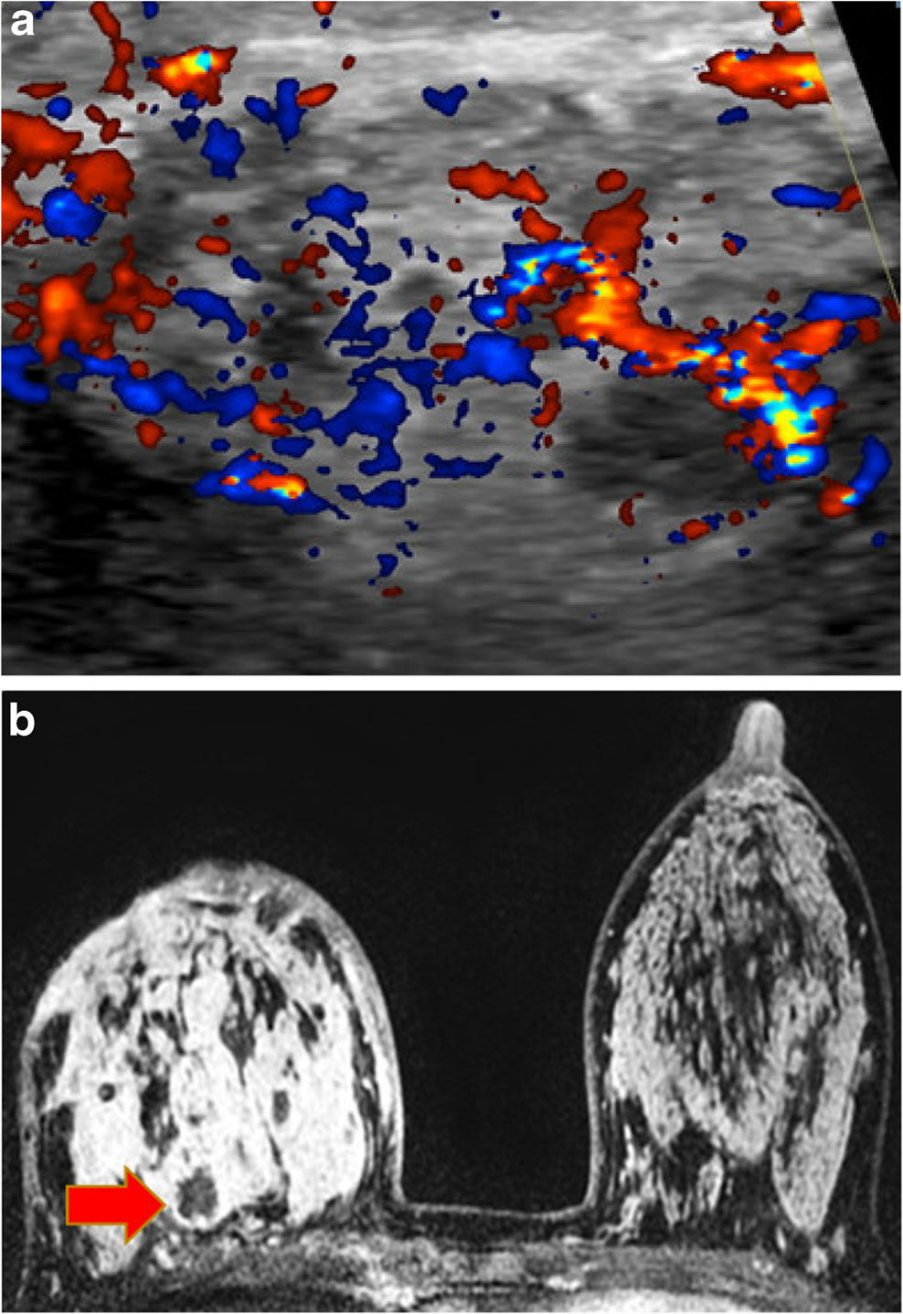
Infectious cause: *Corynebacterium*. A 31-year-old pregnant woman had a red and swollen right breast. On ultrasound-guided biopsy and aspiration, *Corynebacterium* infection was identified. a Ultrasound of the right periareolar region demonstrates marked vascularity on colour Doppler with heterogeneous breast tissue and areas of hypoechogenicity consistent with micro-abscesses (*red arrow*). Appearances are identical to idiopathic granulomatous mastitis sonographically. b Axial contrast-enhanced MRI showed global asymmetry, skin thickening, and diffuse increased enhancement in right breast with non-enhancing areas consistent with small abscess collections (*red arrow*)
